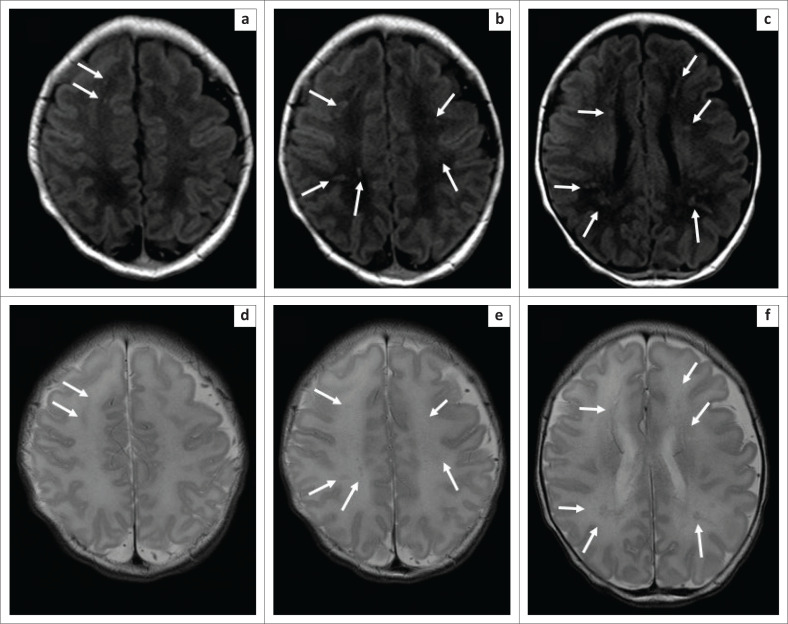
Axial unenhanced T1-weighted MRI of the brain (repetition time msec/echo time msec, 300/2.52, 3-mm section thickness) at the level of centrum semiovale and corona radiata (a, b, c) with corresponding axial T2-weighted MRI of the brain (repetition time msec/echo time msec, 3680/90, 3-mm section thickness) (d, e, f). There were areas of T1 and T2 shortening following the distribution of deep medullary veins (solid white arrows on all images), more evident on the T1-weighted images.
